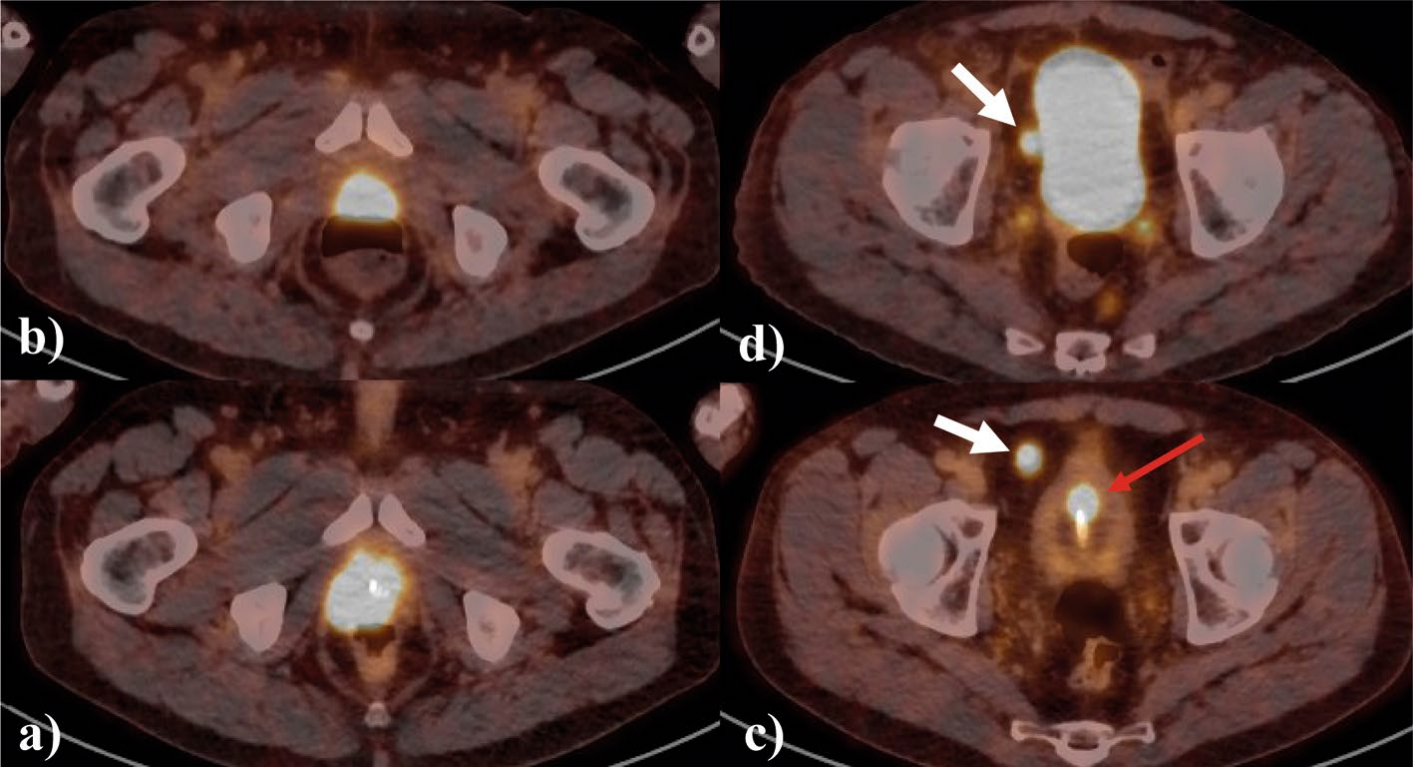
Patient 01 a prostate lesion on pre-surgery PSMA PET/CT; b prostate bed residual lesion on post-surgery PSMA PET/CT; c para-vesical lymph node on pre-surgery PSMA PET/CT; d para-vesical lymph node on post-surgery PSMA PET/CT (white arrow); red arrow represents a lesion in urinary bladder wall
